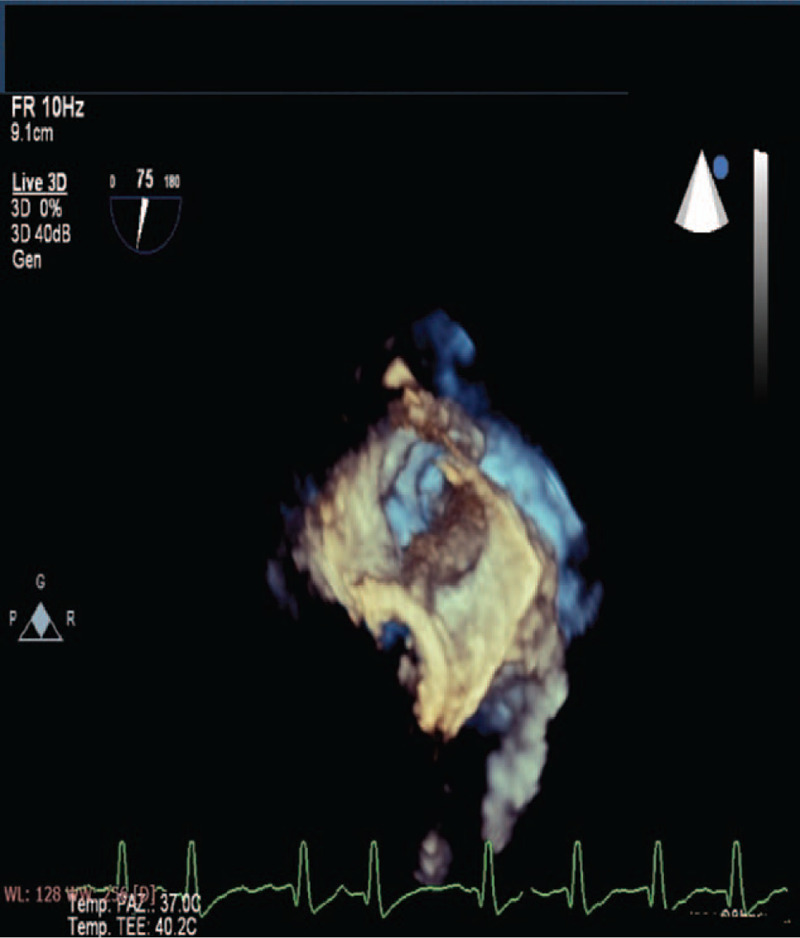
Three-dimensional transesophageal echocardiography showing a left atrial appendage almost entirely occupied by thrombotic material.
